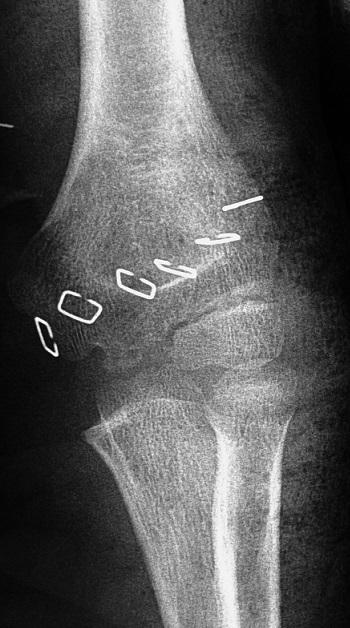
Elbow radiograph after reduction (face)
